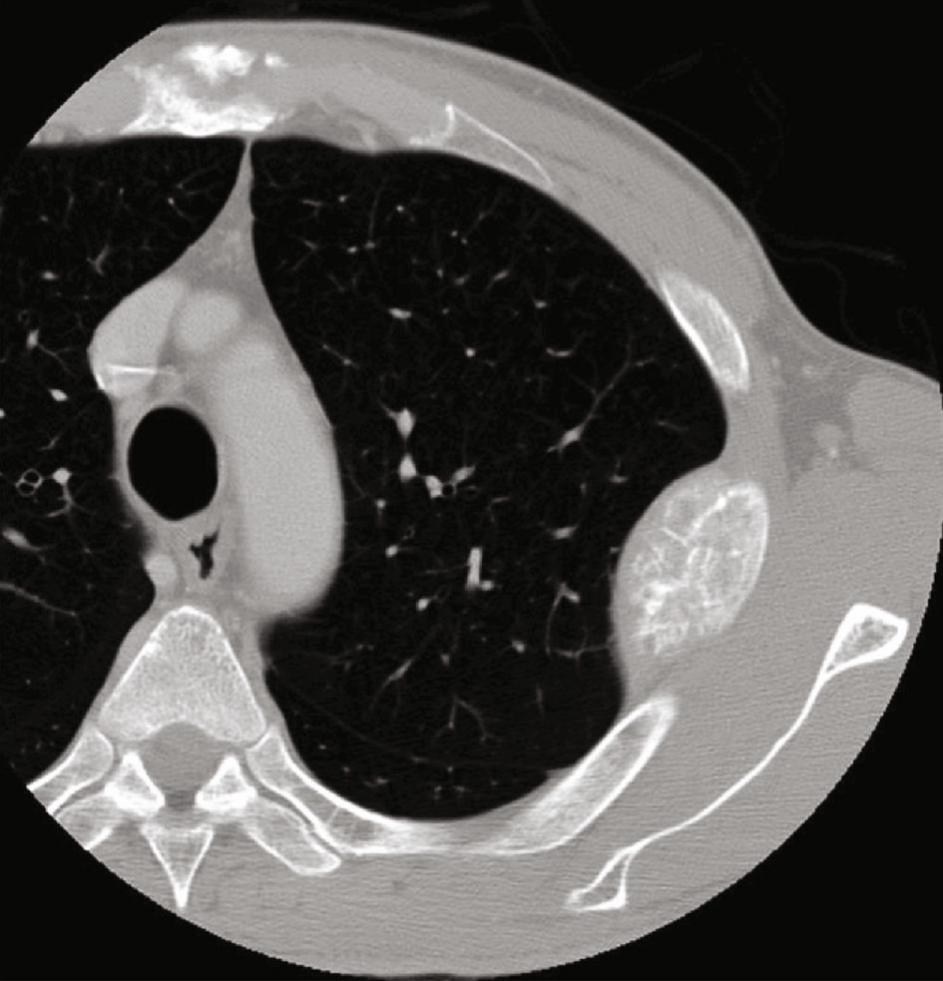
Computed tomography showing a tumor with cortical destruction and honeycomb-like calcifications in the 4th rib.
